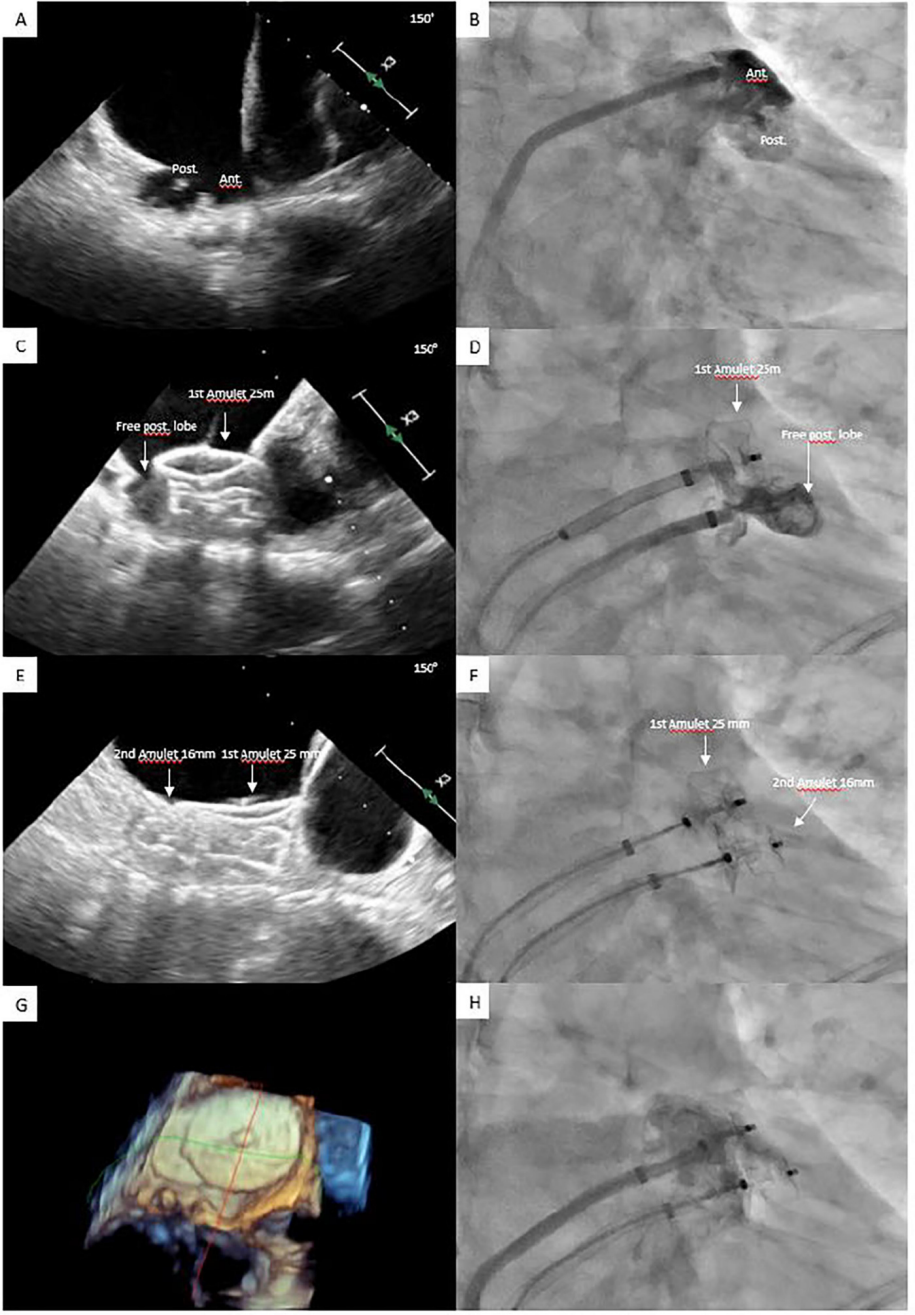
Percutaneous LAAC with concomitant implantation of two LAAC devices by using the double-sheath technique. Large bilobed LAA showed by TEE (A) and angiography (B). Residual patent lobe after implantation of Amulet 25 mm showed by TEE (C) and angiography (D). Complete sealing of bilobed LAA after implantation of a second Amulet device (E–H). LAA, left atrial appendage; LAAC, left atrial appendage closure; TEE, Transesophageal echocardiography.
